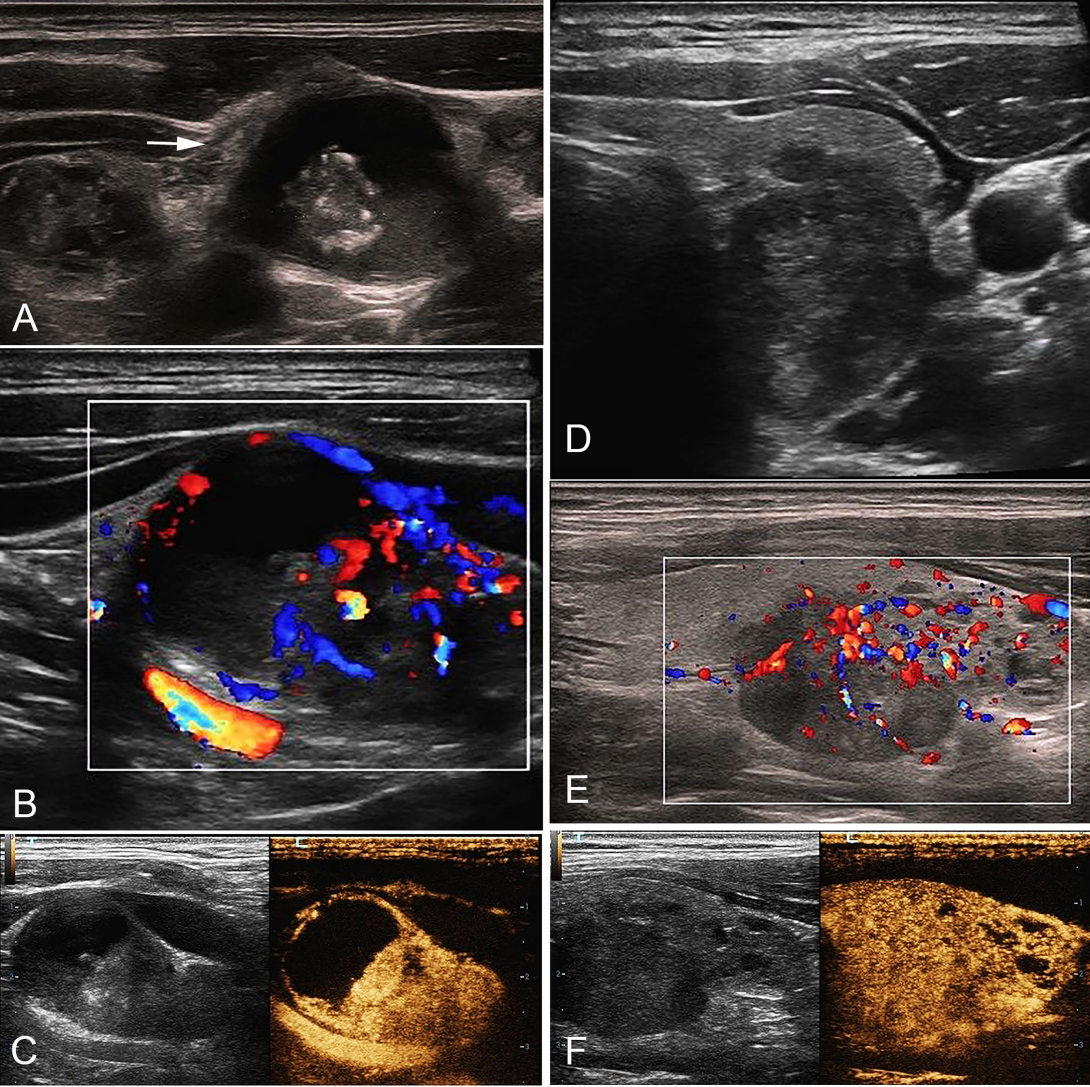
A 35-year-old male with cN1 PTC. (A) Sonogram of the largest metastatic lymph node (arrow) in level IV indicates irregular margin, loss of hilum, calcification, hyper-echogenicity, and cystic change in the node. (B) CDFI shows non-hilar vascularization. (C) CEUS indicates heterogeneous hyper-enhancement and perfusion defect in the node. (D) Sonogram of the PTC lesion shows a solid hypo-echogenic nodule with irregular margin and a taller than wide shape, measuring 1.2*1.4*1.9cm. (E) CDFI indicates abundant blood flow signals. (F) CEUS shows that the PTC lesion presents pervasive hyper-enhancement. PTC, papillary thyroid carcinoma; cN1, clinical N1; CDFI, color doppler flow imaging; CEUS, contrast-enhanced ultrasound.
